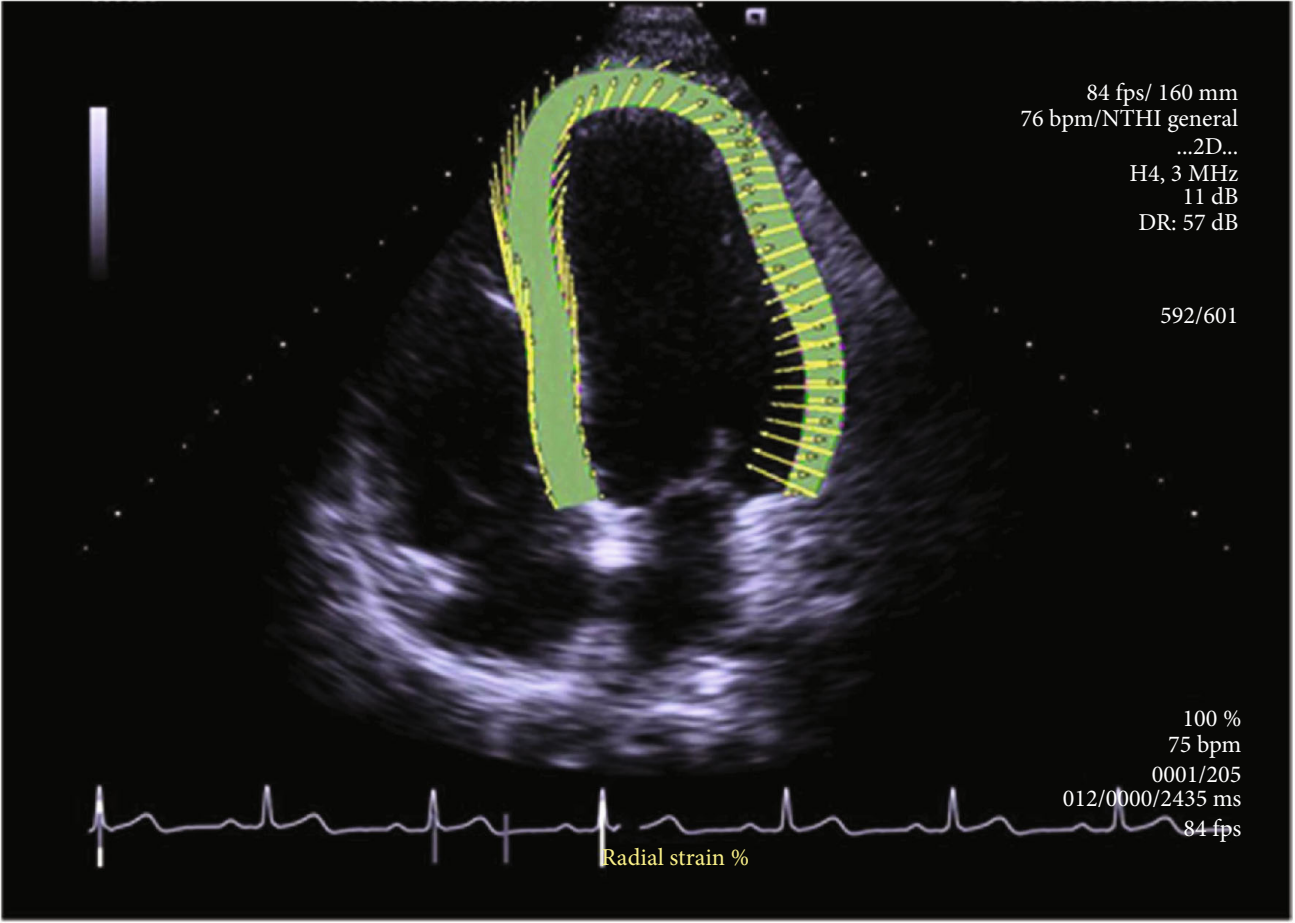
VVI was used to analyze the ventricular apical four-chamber section of the cardiac LS of the endocardium in a healthy individual. Only the left ventricular border was drawn in the corresponding section; the software could automatically generate the LS longitudinal motion direction and displacement and calculate the LS parameters.
